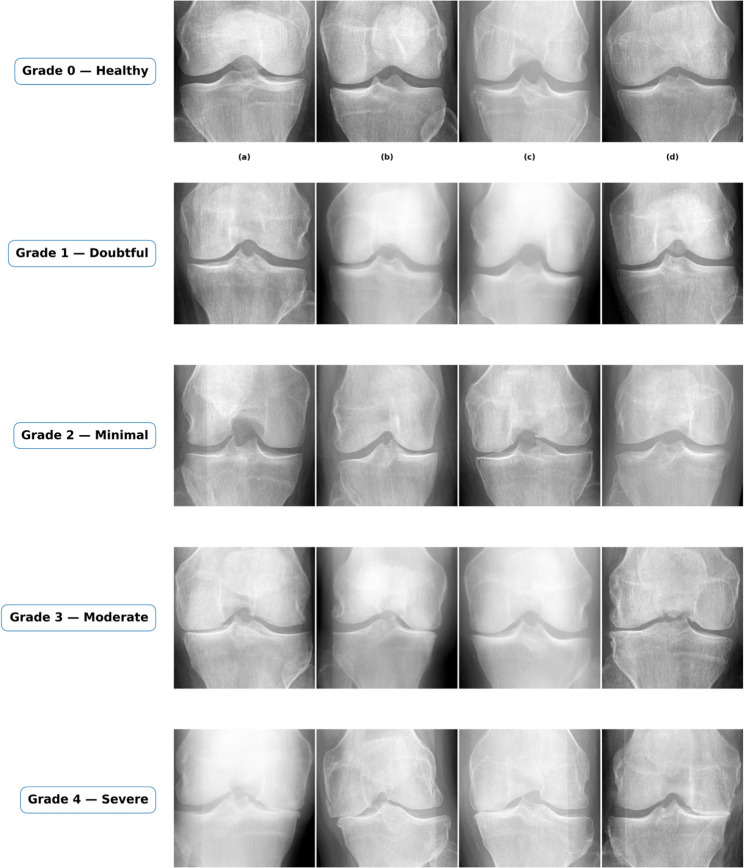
Representative anteroposterior knee radiographs demonstrating the five Kellgren-Lawrence grades (grade 0 to grade 4)
